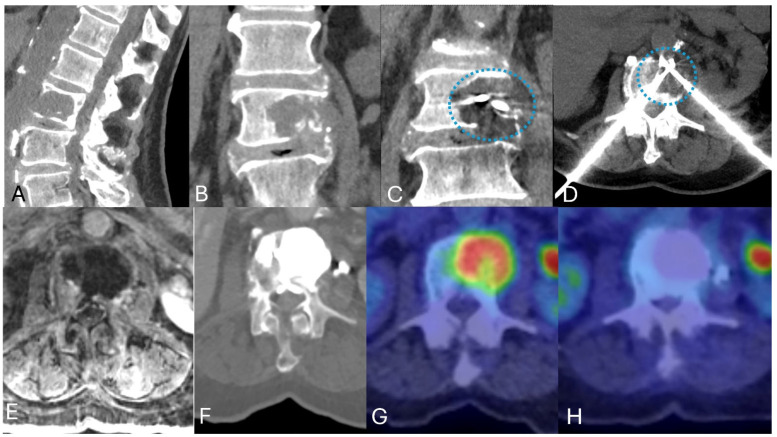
Cryoablation for curative intent. An 82-year-old female smoker with a history of previously radiated L2 metastasis presented with new onset lumbar pain 1 year after radiation. Studies demonstrated worsening of her L2 metastasis and a painful pathologic fracture. (A,B) Sagittal and coronal CT demonstrate a pathologic fracture through a lytic metastasis. (C,D) Coronal and Axial MIP images demonstrate two variable cryoablation probes crossing in the lesion and associated ice ball (dashed circle) at 8 min. (E) Post Gadolinium T1WI with fat saturation demonstrates the thermal margin 3 months after the procedure. (F) Axial CT demonstrates complete filling of the large lytic defect with PMMA cement, providing structural support. (G,H) Axial FDG PET/CT prior to treatment and 1 year after ablation.
